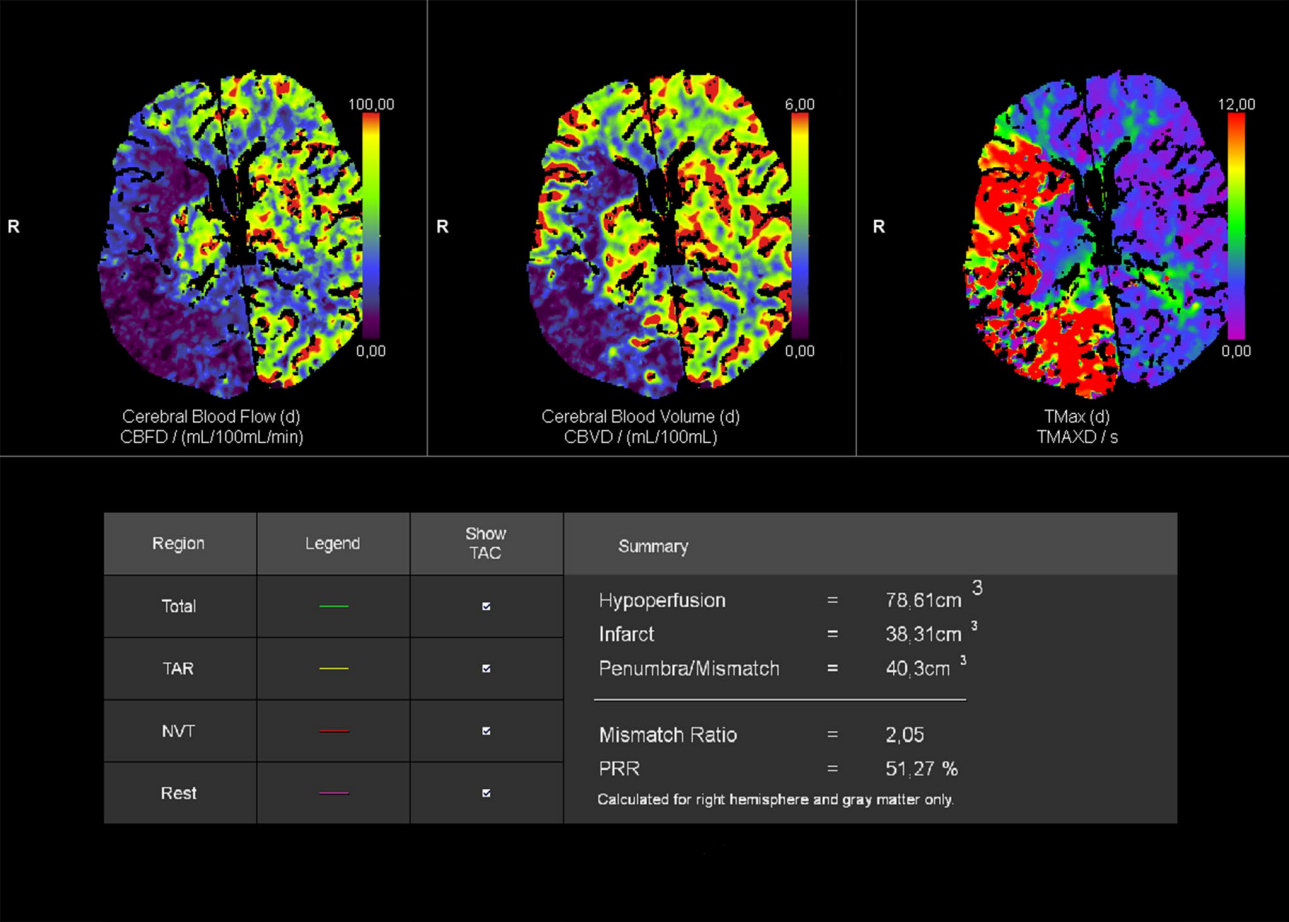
Representative case of a CT perfusion scan analyzed with syngo.CT Neuro Perfusion software. From left to right, the different color-coded perfusion maps depict cerebral blood flow, cerebral blood volume, and Tmax (time to maximum of the residue function), respectively, showing abnormal perfusion in the affected right brain hemisphere. Below, the table summarizes automatically calculated perfusion volumes and ratios, including the infarct core volume (38.31 cm³)
